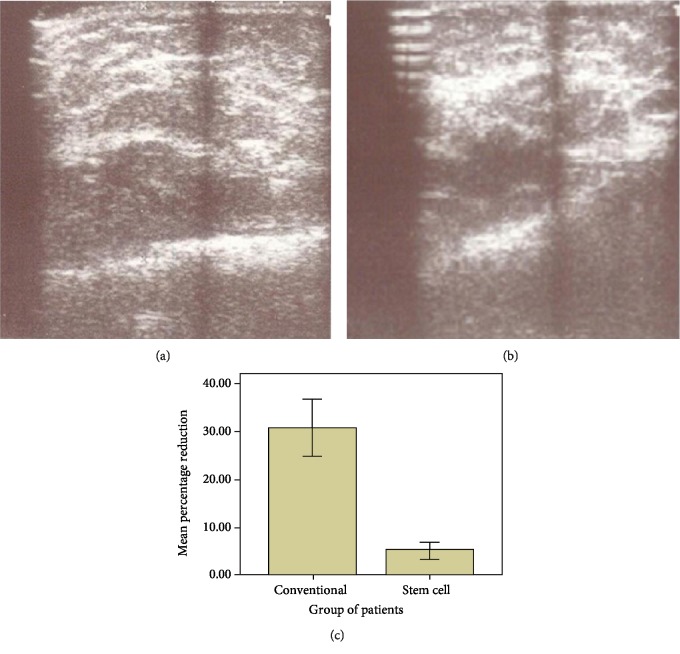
Representative ultrasonic measurement of soft tissue thickness (a) after 72 hours and (b) 6 months after transplantation. (c) Comparison of mean percentage reduction of fat absorption in both groups six months after the 1^st^ fat graft session.
